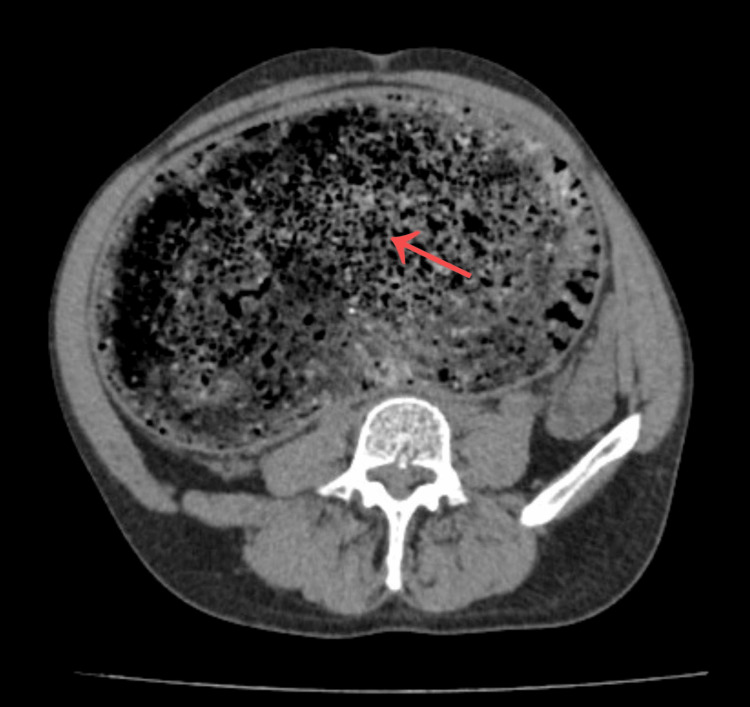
Axial CT demonstrating sigmoid fecaloma. Axial contrast-enhanced computed tomography (CT) image showing marked colonic dilation with a well-defined, heterogeneous intraluminal mass with a mottled gas pattern in the sigmoid colon (arrow), consistent with fecaloma causing distal large bowel obstruction.
